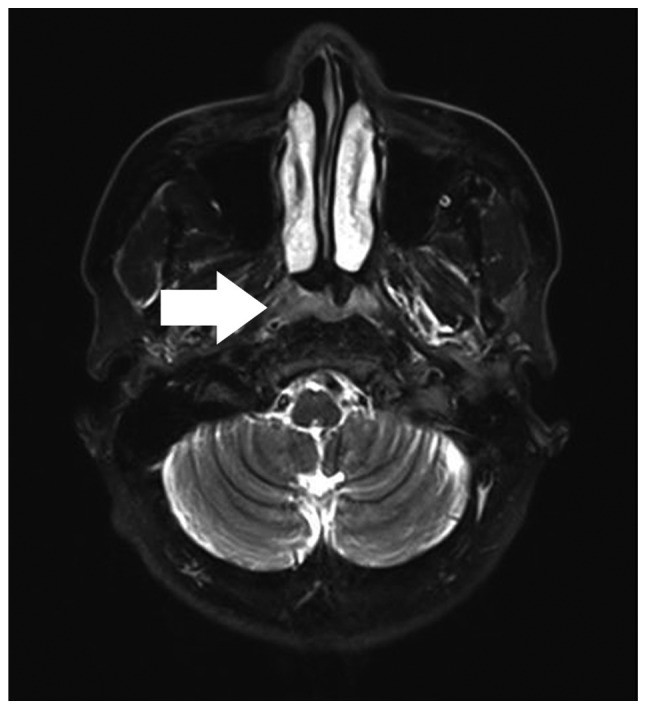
Initial magnetic resonance imaging (MRI) scan of the nasopharynx showing soft-tissue thickening of the top wall of the nasopharynx (arrow).
